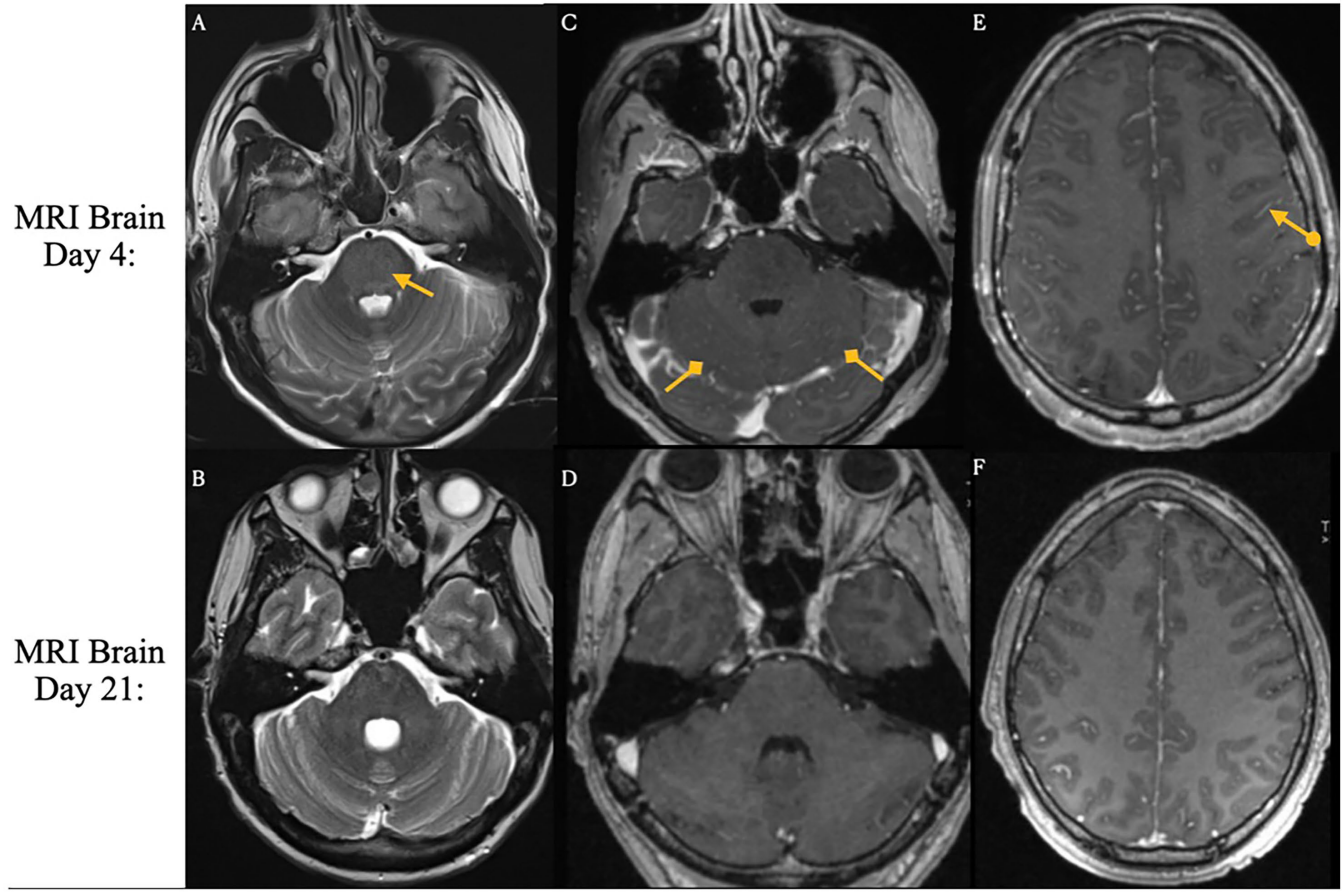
Brain magnetic resonance imaging on days 4 and 21 following CAR T-cell infusion. ICANS-associated brain MRI findings. T2 and FLAIR sequences reveal hyperintensity throughout the cortex and pons, accompanied by diffuse leptomeningeal enhancement. Images were acquired pre-treatment (Day 4 Panels A,C,E) and post-treatment (Day 21. Panels B,D,F) following steroid and Anakinra therapy.
